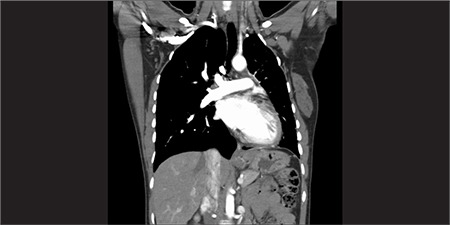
Coronary oriented maximum-intensity-projection image from CT angiography shows small left hemithorax and absence of left main pulmonary artery. Note the left subdiaphragmatic collateral branches
